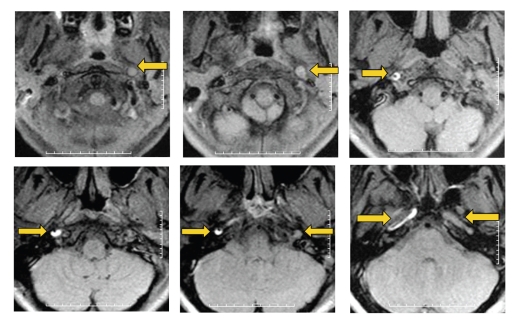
MRI T1-FAT-SAT-weighted images showing the dissecting process visible as a hypersignal in the wall of both ICAs as well as an enhancement of ICA diameter (arrows).
